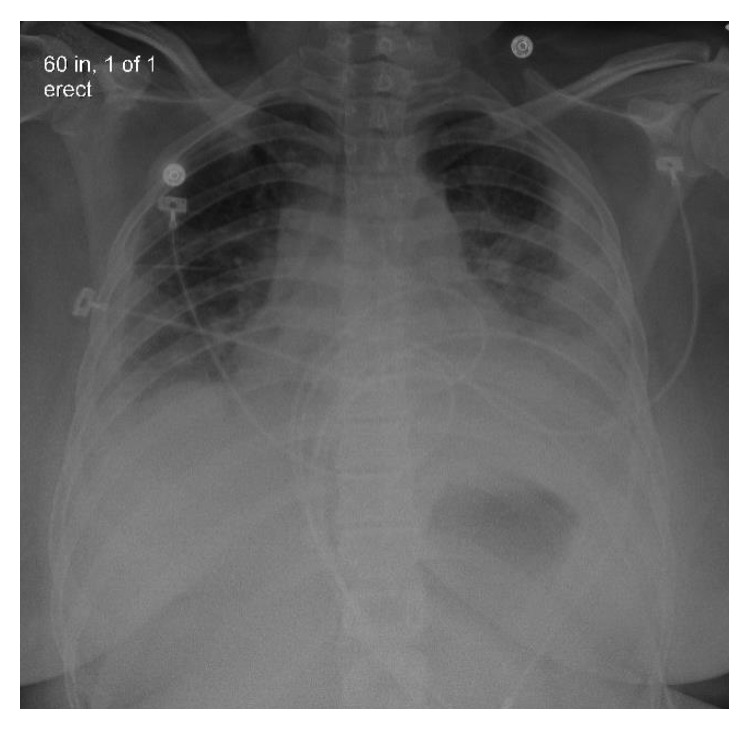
Interval chest X-ray showing worsening bilateral effusions.
